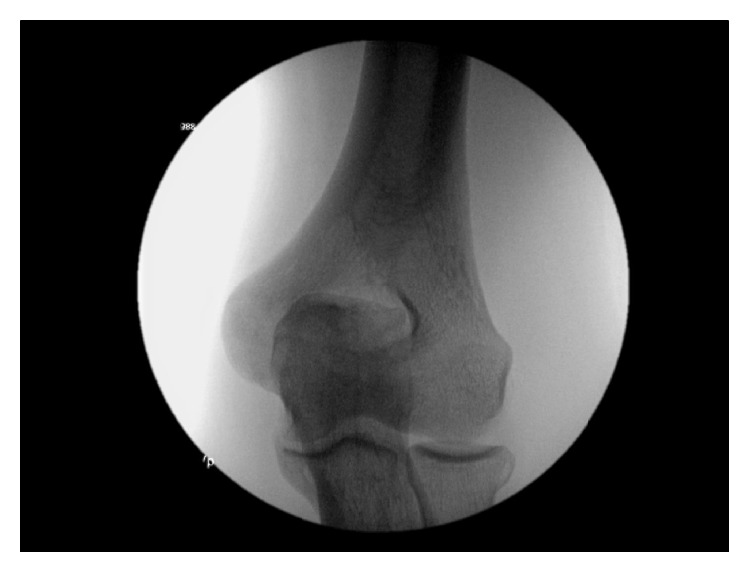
Intraoperative radiograph. Intraoperative stress view of the left elbow demonstrating valgus laxity.
